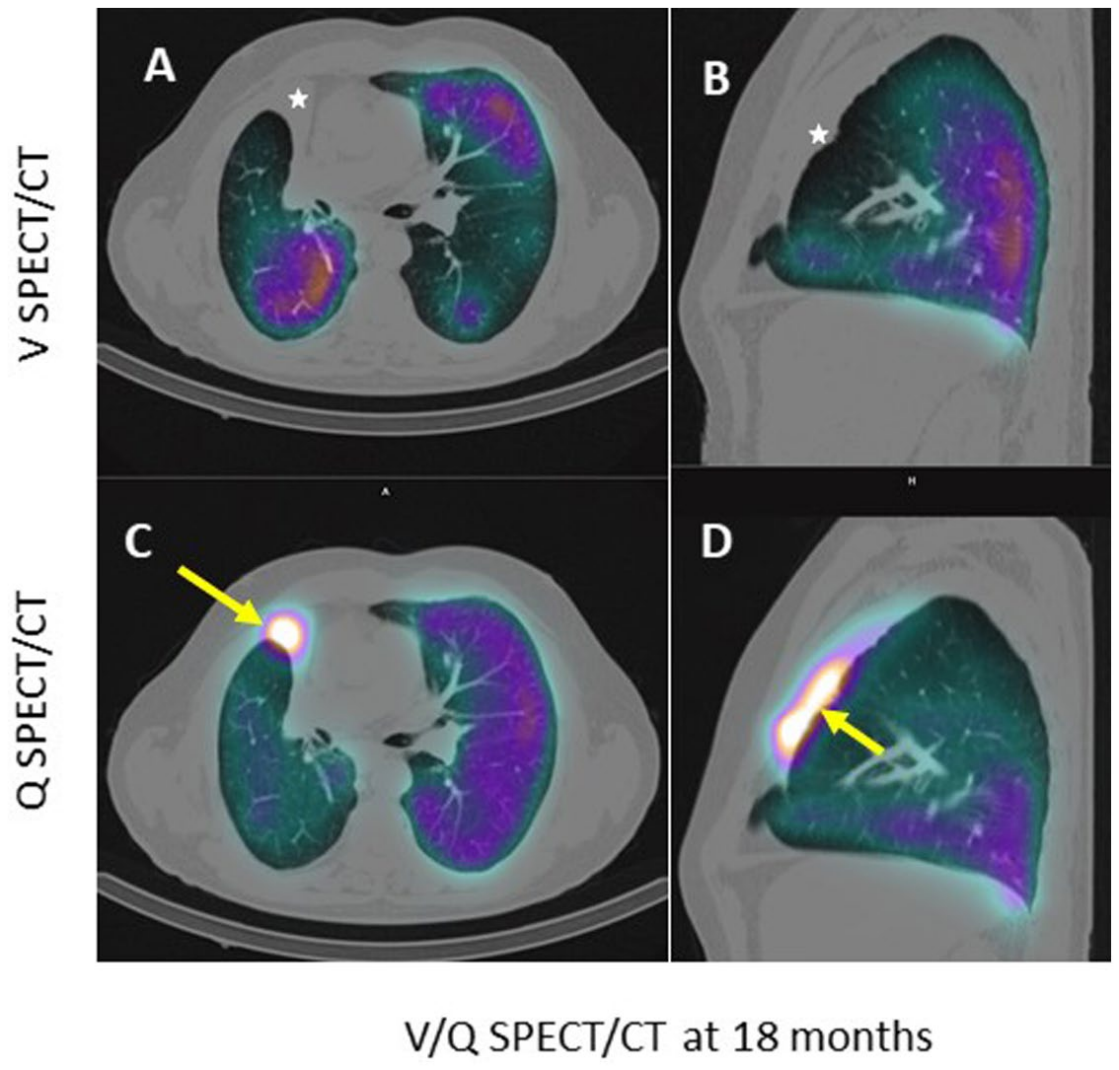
Axial (A–C) and sagittal (B–D) CT slices passing through the atelectasis, in pulmonary window. The white arrow shows the very high uptake of macroaggregated albumin in the perfused atelectasis (C,D); the white star shows the absence of ventilation (A,B).
